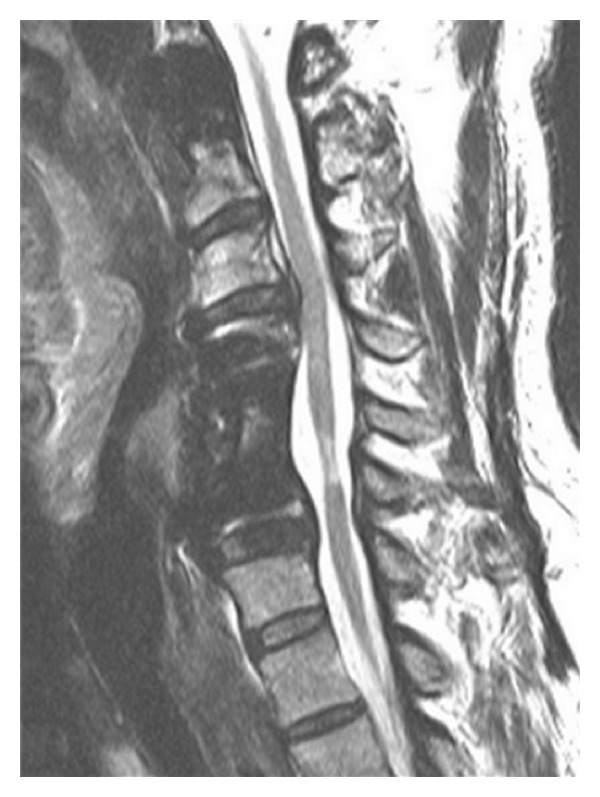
Sagittal T2-weighted MRI at the latest 16-month followup showing a persistent “white cord syndrome.”
